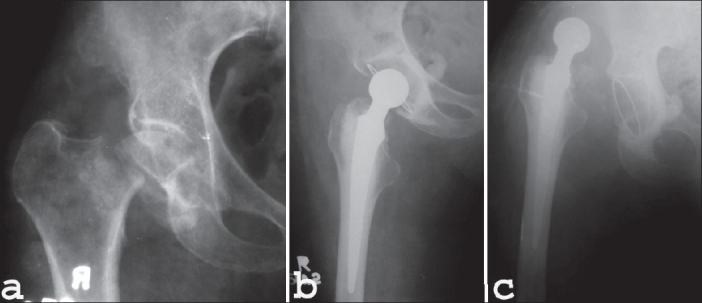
Antero-posterior radiograph (a) of 60 years female with Garden stage IV and Pauwels type II subcapital femoral neck fracture. Immediate postoperative antero-posterior radiograph (b) of the same patient after total hip arthroplasty. Antero-posterior radiograph (c) at 3 weeks showing dislocation of the prosthesis. The acetabular component tilted vertically from its initial position. Poor cementing of the acetabular component resulted in dislocation of the hip
